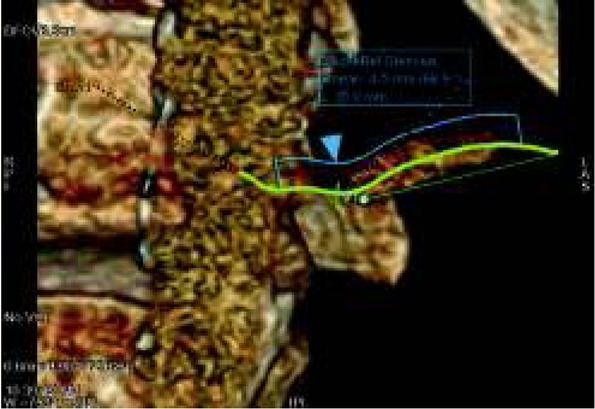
CT Angiography. Left renal artery stenosis. Measurement of arterial stenosis. Ryc. 6. Angio CT. Zwężenie lewej tętnicy nerkowej w miejscu odejścia od aorty, pomiar zwężenia światła naczynia.
